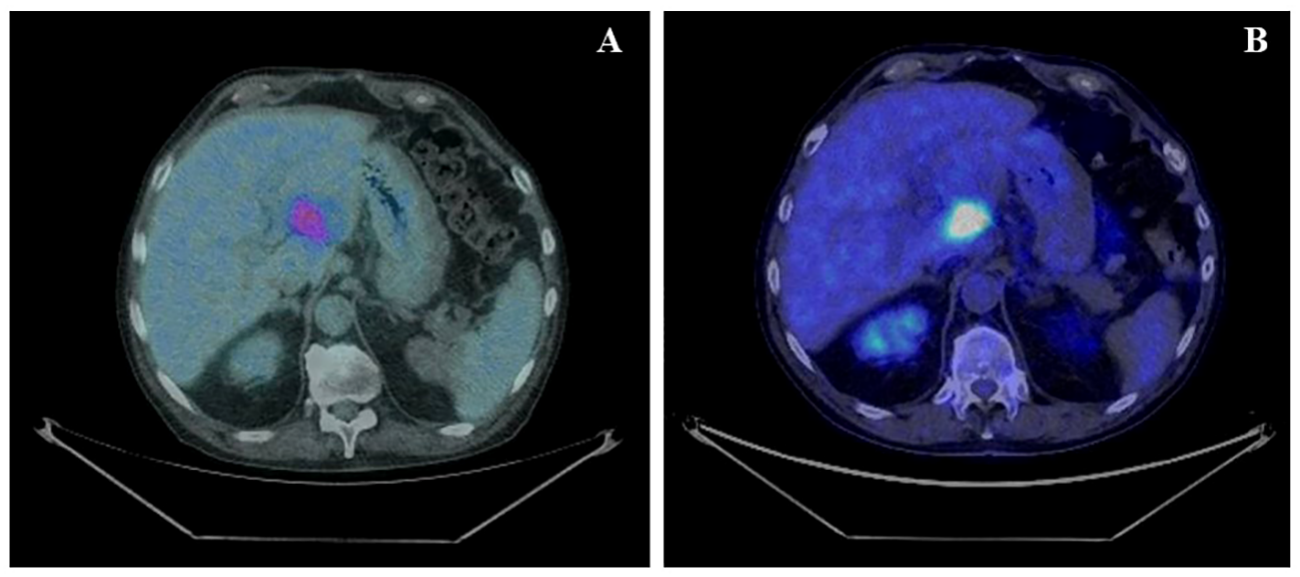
(A) Basal axial PET/CT fused images, showing a hypermetabolic lesion involving II–III liver segments (SUVmax 9.4). (B) Axial PET/CT fused images, restaging scan after a month of imatinib therapy: the hypermetabolic area appears increased in size and shows a greater ^18^F-FDG uptake: SUVmax 20.1 (vs. 9.4).
